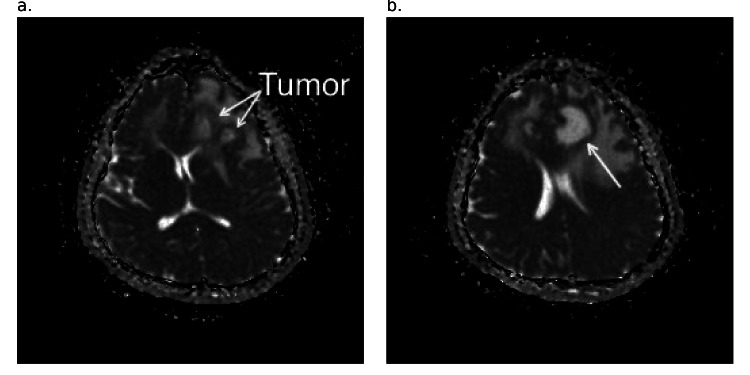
Axial ADC map MRI image a. At the level of the capsuloganglionic region b. At the level of the body of the lateral ventricles, a peripherally enhancing tumor(white arrow) ADC: apparent diffusion coefficient; MRI: magnetic resonance imaging
